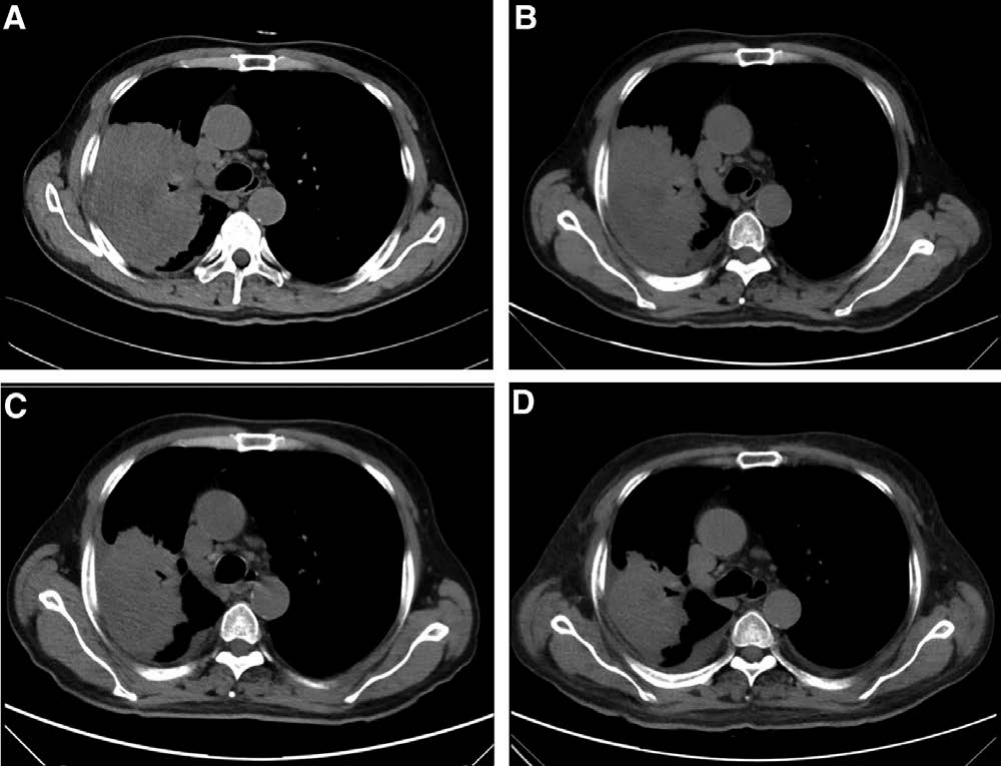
Chest CT images obtained following treatments (mediastinal window). At diagnosis (A), day 21 (B) after a cycle of chemotherapy with pemetrexed and carboplatin, the 28th day (C) and 59th day (D) after given a cycle of camrelizumab plus pemetrexed in combination with carboplatin. CT = computed tomography.
